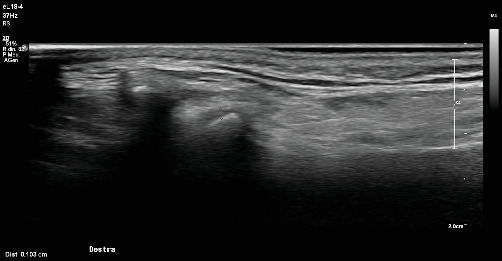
TMJ ultrasound: Erosion and flattening of the condylar head in RA—coronal scan, the flattening is visible between the two pointers in the viewer.
